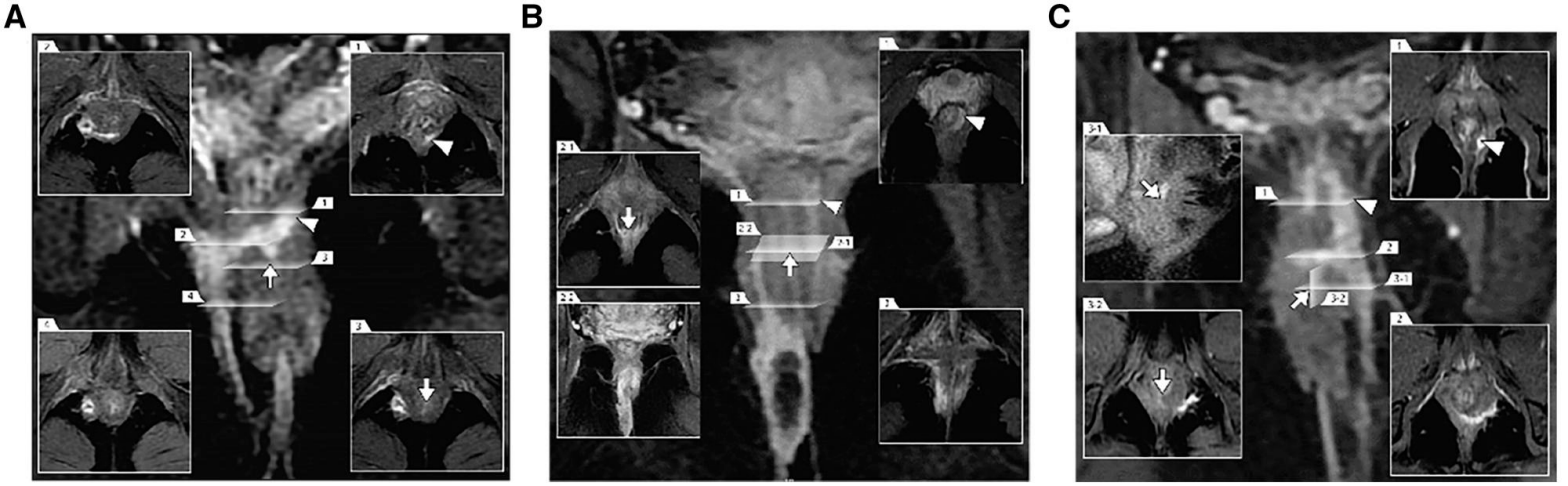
Different types of posterior complex anal fistulas from our cohort, as seen on coronal contrast-enhanced fat-suppressed T1-weighted images with different corresponding sections. (A) The suprasphincteric anal fistula with multiple branches in the deep intersphincteric horseshoe tracts and right ischiorectal fossa. (B) The low transphincteric anal fistula with right ischiorectal fossa abscess and deep intersphincteric horseshoe tracts, extending along the left combined longitudinal muscle into the supralevator area of the rectal muscle. (C) The high transsphincteric anal fistula in the left puborectal muscle with up spread supralevator into the pelvirectal space. The arrows are pointing at the IO. The triangles represent deep branches. IO = internal opening.
